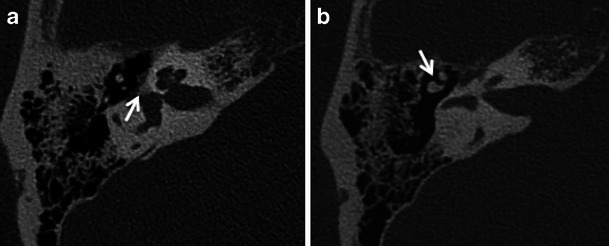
a Axial HRCT image of the right temporal bone in an adult patient with progressive right-sided CHL and remote history of ipsilateral head injury. A hypodense demineralised otosclerotic plaque (*arrow*) is noted in the fissula ante fenestram. b Axial HRCT image of the same patient as (a) at a slightly higher level. There is also evidence of malleo-incudal dislocation (*arrow*)
